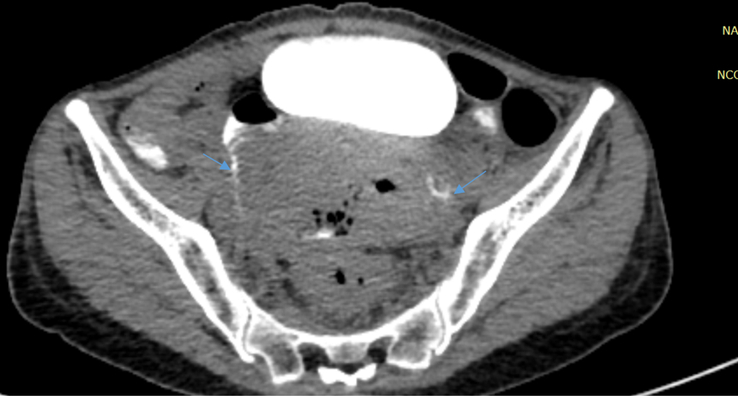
Axial section of computed tomography (CT) of abdomen and pelvis with rectal contrast showing thin rim of contrast passing from rectum to jejunal loops on bilateral sides via the fistulas (thin blue arrows).
